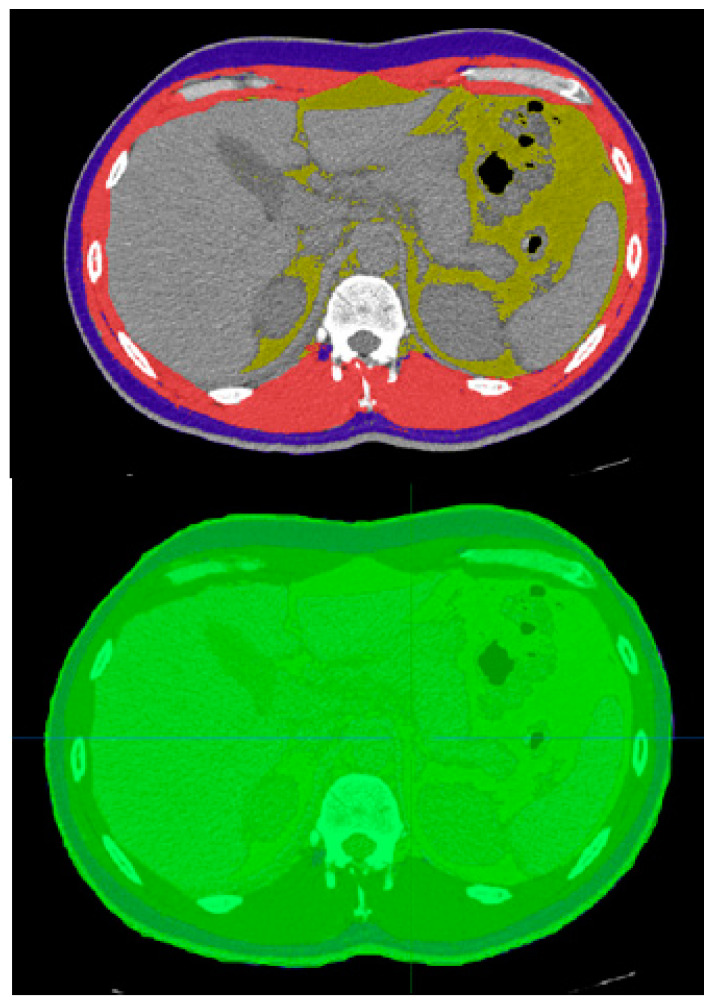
The red, blue, and yellow areas (upper image) are total muscles, subcutaneous fat, and visceral fat, respectively, in this section. The total body volume in the mentioned CT cut (i.e., the volume of total body cross-section in this slice (lower image, green area)) was measured, then the muscle and fat volume ratio to total body volume was calculated to cancel out the effect of any possible zoom-out or magnification on the image.
